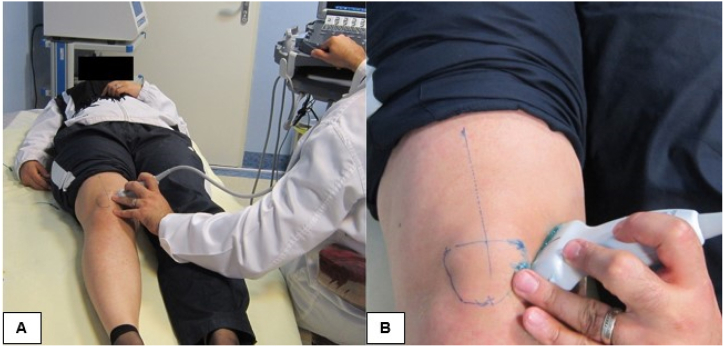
Muscle imaging technique with ultrasound. (a) The subjects were placed supinely on a bed with both lower limbs in neutral position and knees in full extension. (b) The medial (vastus medialis) and lateral (vastus lateralis) patellar aspects were scanned.
